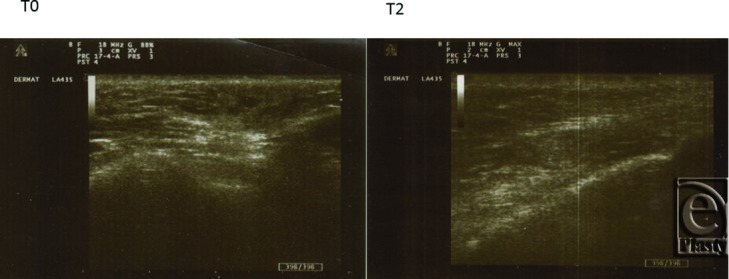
Malar area. *T*_0_: Evident widening of the deep dermal thickness, which is nonhomogeneous, with multiple echo scattering. *T*_2_: In this area, the collagen volume is not amplified, but the tissue hydration renders homogeneous the background, with reduced ultrasound beam reflection.
